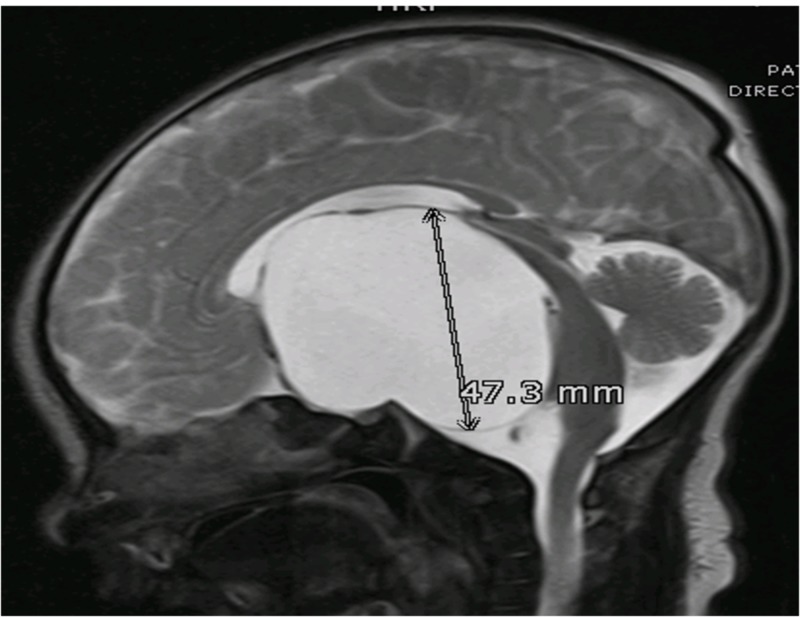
MRI T2WI, Sagittal Section A large extra-axial, well-defined CSF intensity cystic lesion is identified in the midline in the sellar and suprasellar region. It measures 48 x 62 x 47 mm in AP transverse and cranio-caudal dimension. This lesion is causing a compressive effect on the third ventricle and bilateral lateral ventricles. These findings are consistent with arachnoid cyst. MRI T2WI: magnetic resonance imaging T2 weighted image; CSF: cerebrospinal fluid.
